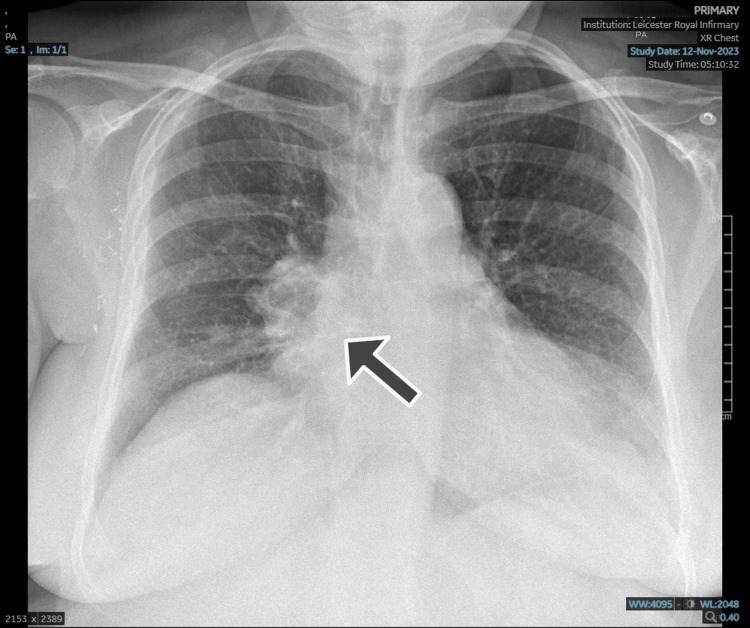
Initial chest X-ray Posteroanterior chest radiograph showing a spiculated opacity in the right mid-zone (black arrow), suggestive of a pulmonary mass. The lesion projects over the right hilum and may represent a primary bronchogenic carcinoma. No gross pleural effusion or pneumothorax is identified.
